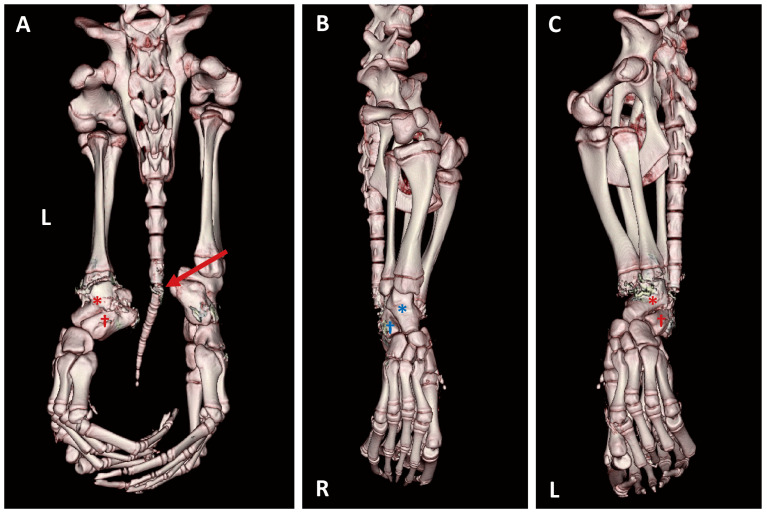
Three-dimensional reconstructed CT images of skeletal injuries. Asterisk (*) indicates the astragalus; dagger (†) indicates the calcaneus. Abnormal findings are indicated in red, whereas the contralateral normal structures are indicated in blue for comparison. Sand artifacts are present around the affected regions. (A) Dorsoventral view showing a distinct coccygeal vertebral fracture (arrow) and subluxation of the astragalus (*) and calcaneus (†). (B) Right lateral view demonstrating normal alignment of the right astragalus (*) and calcaneus (†). (C) Left lateral view showing subluxation of the astragalus (*) and calcaneus (†) with dorsomedial displacement compared to the contralateral side.
